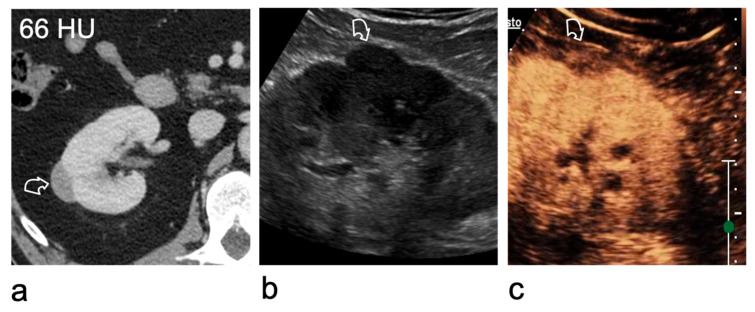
Characterisation of a right renal lesion indeterminate on contrast-enhanced CT. (a) Contrast-enhanced CT acquired only in the nephrographic phase shows a lesion (curved arrow) measuring 66 HU, which cannot be classified as solid or cystic because the unenhanced attenuation is unavailable. (b) On greyscale US, the lesion (curved arrow) remains equivocal as it is isoechoic to the renal parenchyma. (c) CEUS demonstrates that the lesion (curved arrow) is a solid, hypoenhancing tumour. A papillary renal cell carcinoma was found at surgery.
